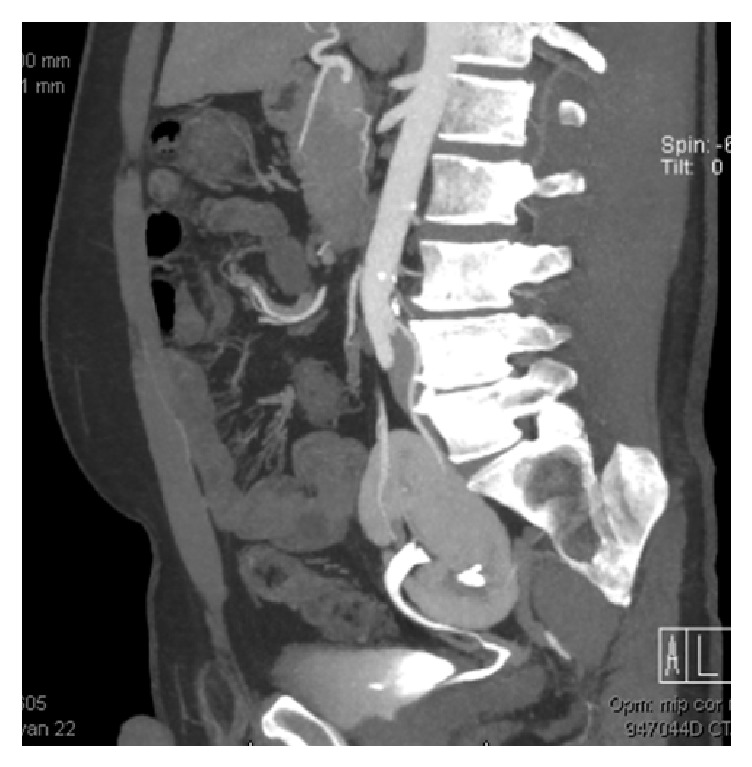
CT scan with sagittal image of ectopic left pelvic kidney showing the upper and middle polar artery.
